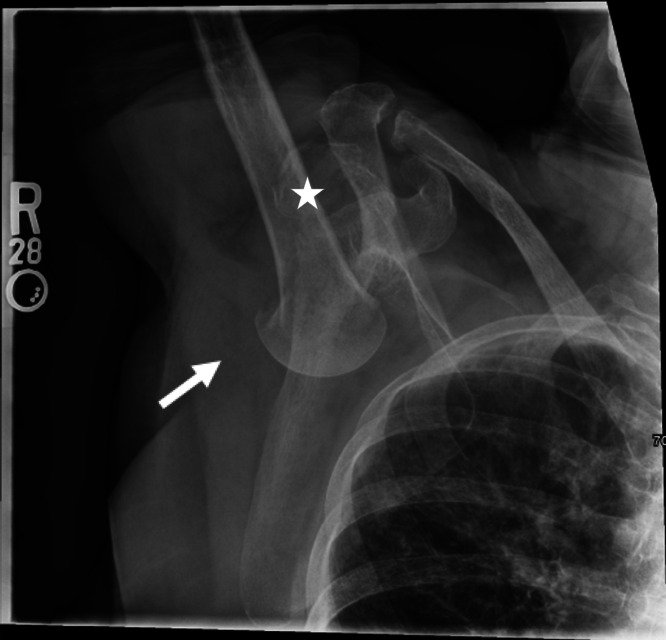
Initial radiograph of right shoulder shows a right inferior dislocation [luxatio erecta] with a concomitant impacted humeral neck fracture (arrow). A mildly displaced right glenoid fracture is also noted (star).
